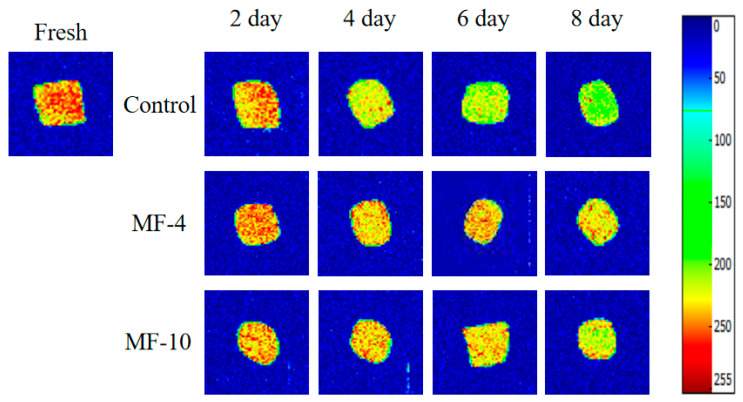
Magnetic resonance images of pork tenderloin at different storage conditions. Control: the storage without magnetic field; MF-4: 4 mT static magnetic field-assisted freezing storage; MF-10: 10 mT static magnetic field-assisted freezing storage.
